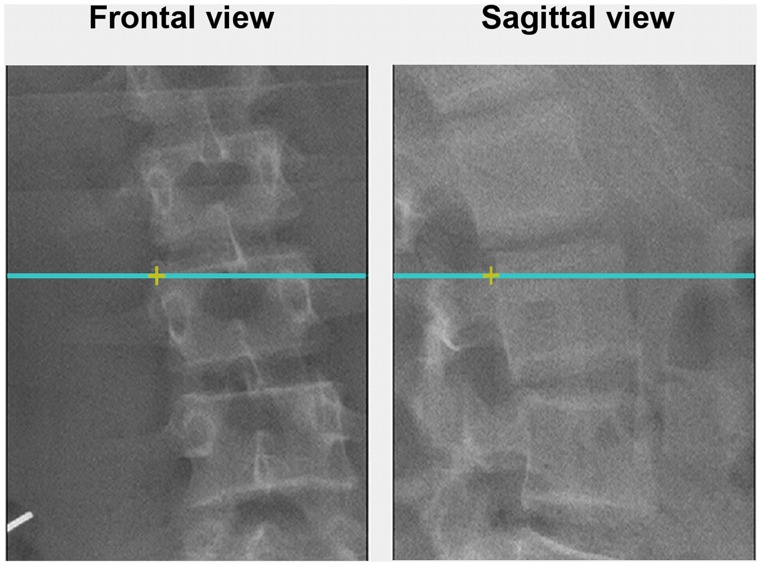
Thoracic and lumbar vertebrae digitization. Firstly a bony landmark was identified on the postero-anterior radiograph. A horizontal line drawn from this point was projected on the lateral radiograph. The intersection between the horizontal line and the edge of the vertebral body ensured that the same bony landmark was identified in each pair of radiographs.
